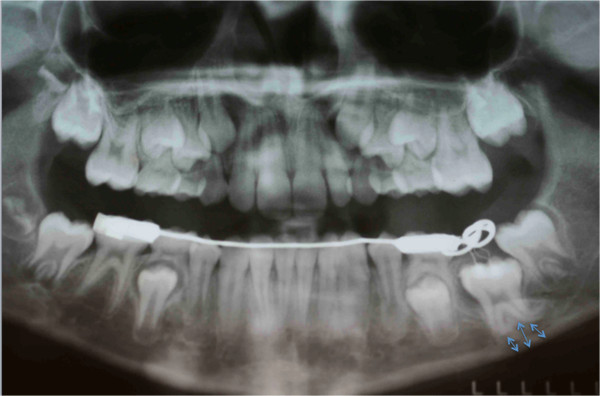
Orthopantomograph 3 months after surgery: it confirmed that the tooth was moving, as evidenced by the increase in the distance between the lower edge of the jaw and the roots of the impacted tooth (blue arrows).
